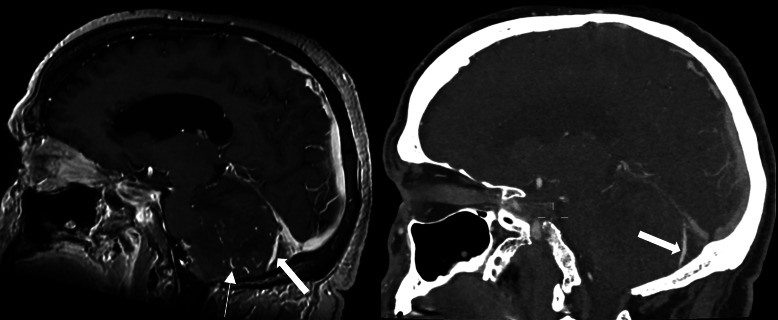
Sagittal magnetic resonance imaging of the brain with T1 images after contrast (left) and sagittal CT imaging of the brain after intravenous contrast (right). Notice the arterial collaterals in the cerebellum (thin arrow) and early enhancement of the inferior vermian vein (thick arrow) suggesting an arteriovenous fistula.
